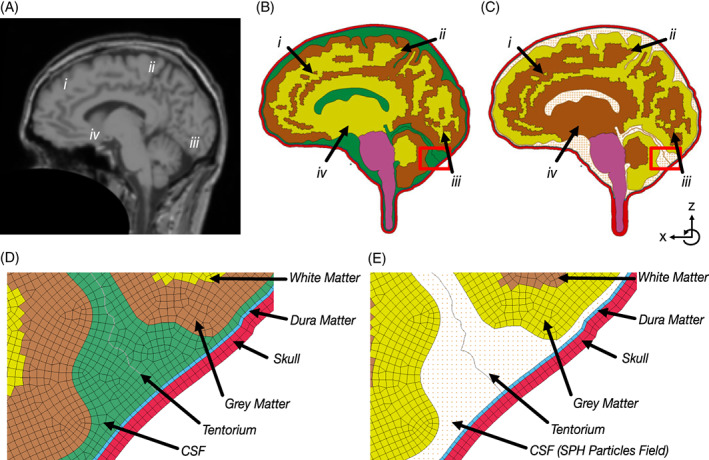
(A) T1 MRI scan of parasagittal plane of subject with approximate data collection locations labelled i–iv, (B) and (C) FE and SPH model with approximate data collection locations labelled i–iv (axes shown in bottom right corner, y axis normal to plane, rotation counter clockwise) (D) and (E) Detail of sulcal and gyral region of FE model and SPH model respectively
